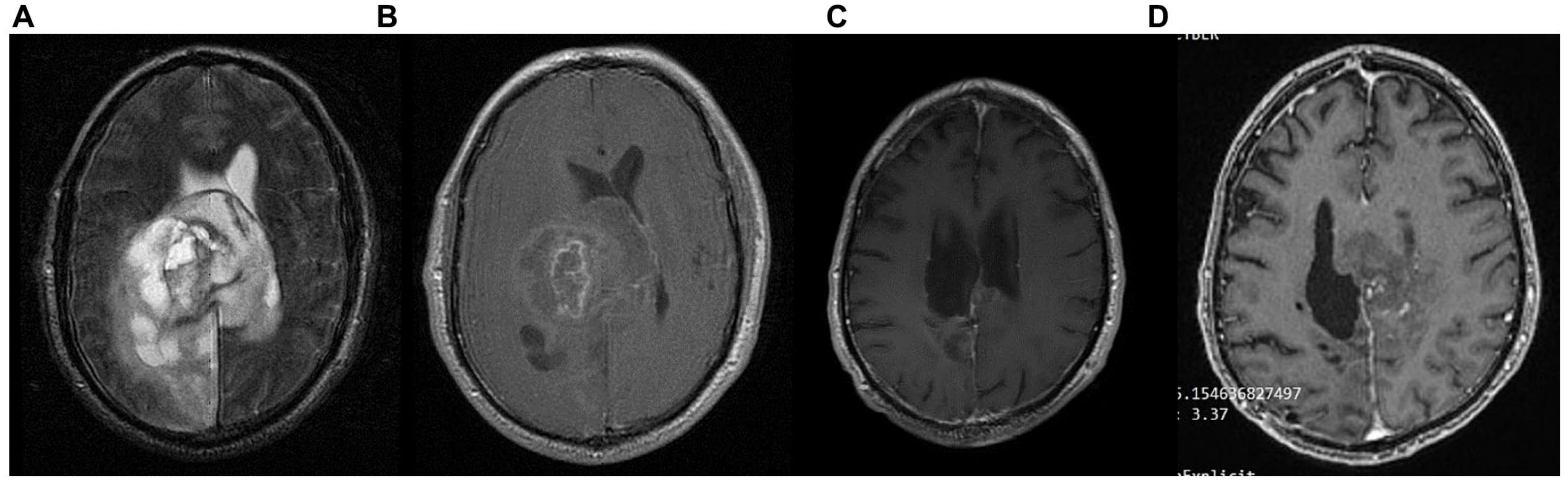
Patient 5: (A) pre-operative brain MRI (T2/FLAIR) (B) pre-operative brain MRI (T1 with contrast) (C) 34-month follow up brain MRI (T1 with contrast) (D) GBM relapse 40-month follow up brain MRI (T1 with contrast).
